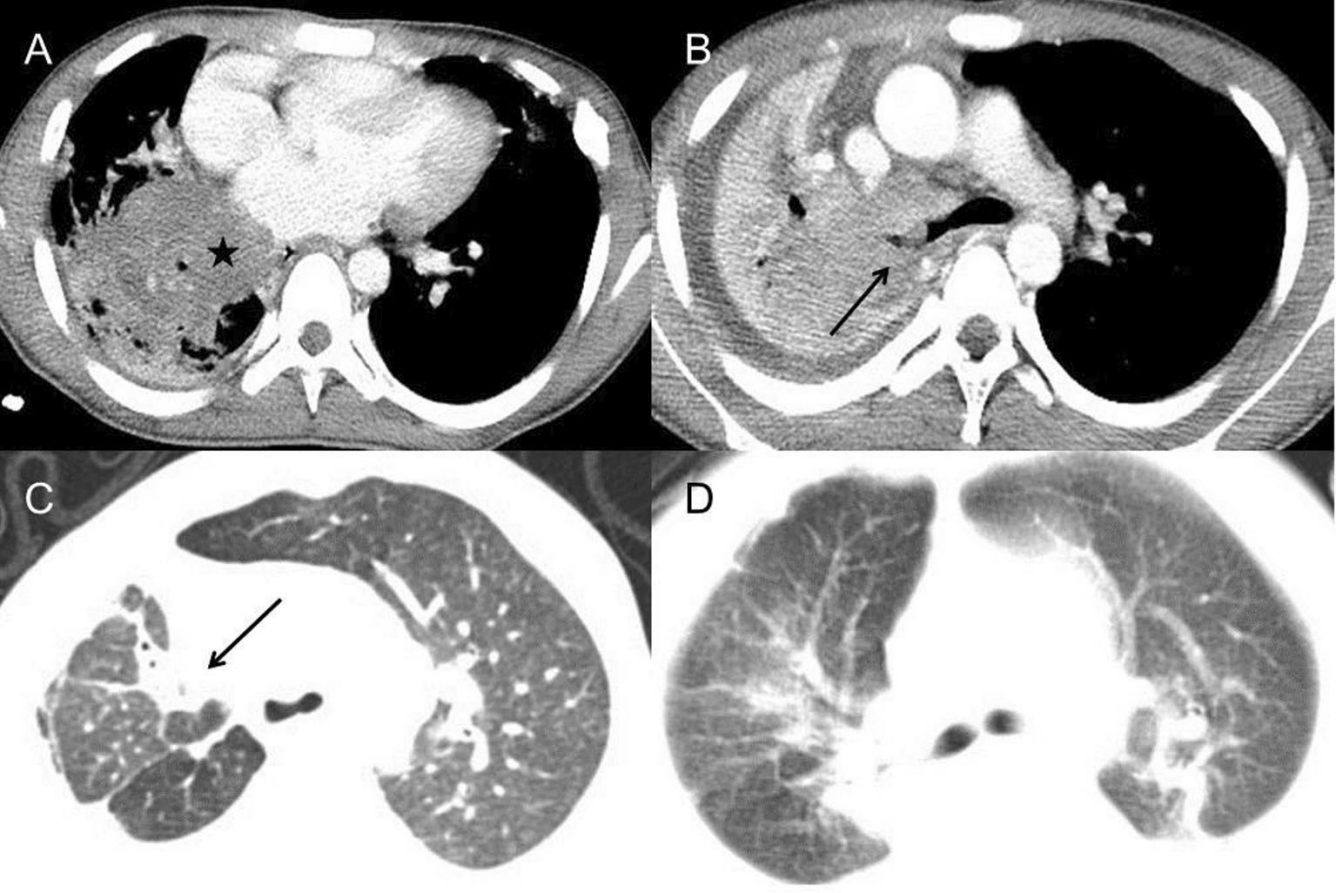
Pulmonary CT of TB infection. (A,B) 16-year-old boy with TB infection. (A) Post-contrast CT showed a large mass in the right lower lobe, about 5 cm in diameter (star). Multiple small cavities were found in the adjacent area of consolidation. (B) A nodule in the right bronchus led to atelectasis of the right lung, which was identified as TB granuloma formation by bronchoscopy (long arrow). The right pleural effusion was also found. (C) In a 3-year-old boy, axial CT scan showed scarring, segmental atelectasis and volume loss in the right upper lobe (long arrow). Slight bronchiectasis could be found inside the area of consolidation. (D) Segmental consolidation in bilateral upper lobes with adjacent ground-glass opacity were found in a 2-year-old boy.
